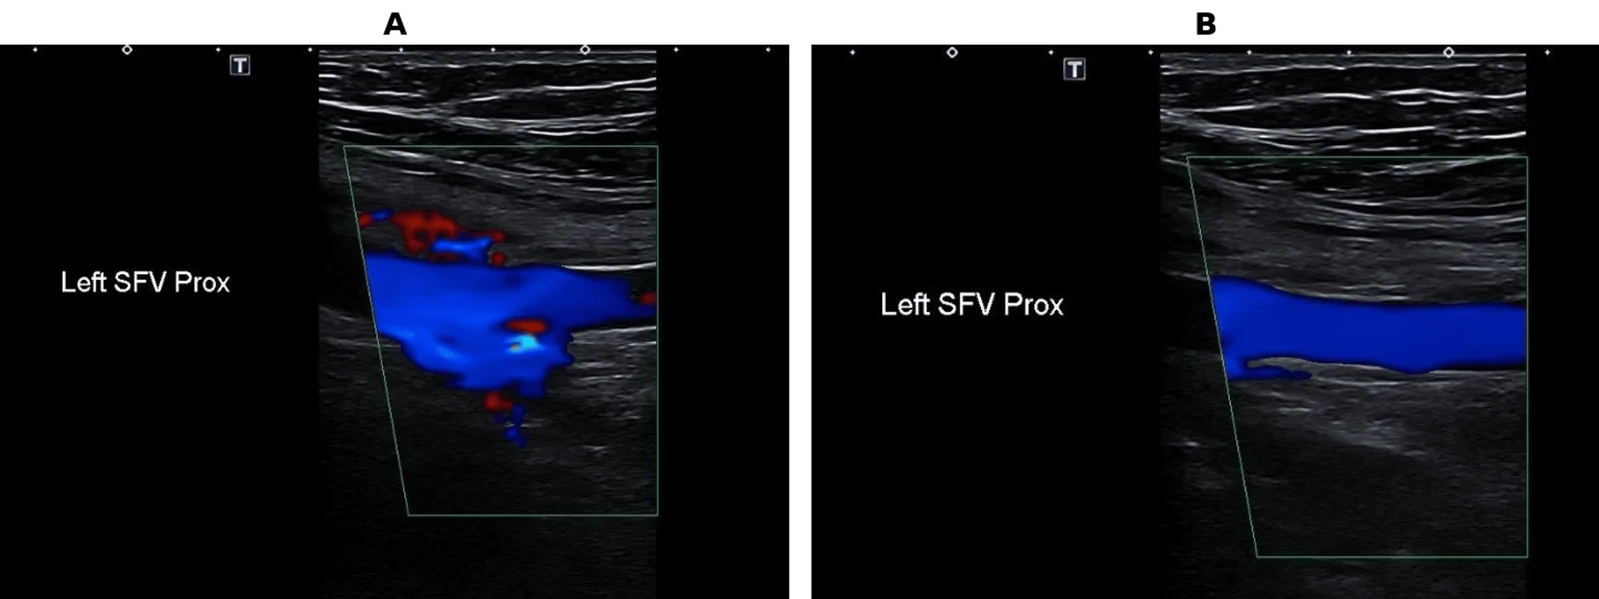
Doppler ultrasound of the left proximal superficial femoral vein (SFV). (A) Color Doppler showing a filling defect with markedly reduced color flow within the vein lumen. (B) Grayscale image with color overlay showing an echogenic intraluminal thrombus with partial flow around it. Both findings are consistent with acute deep venous thrombosis.
